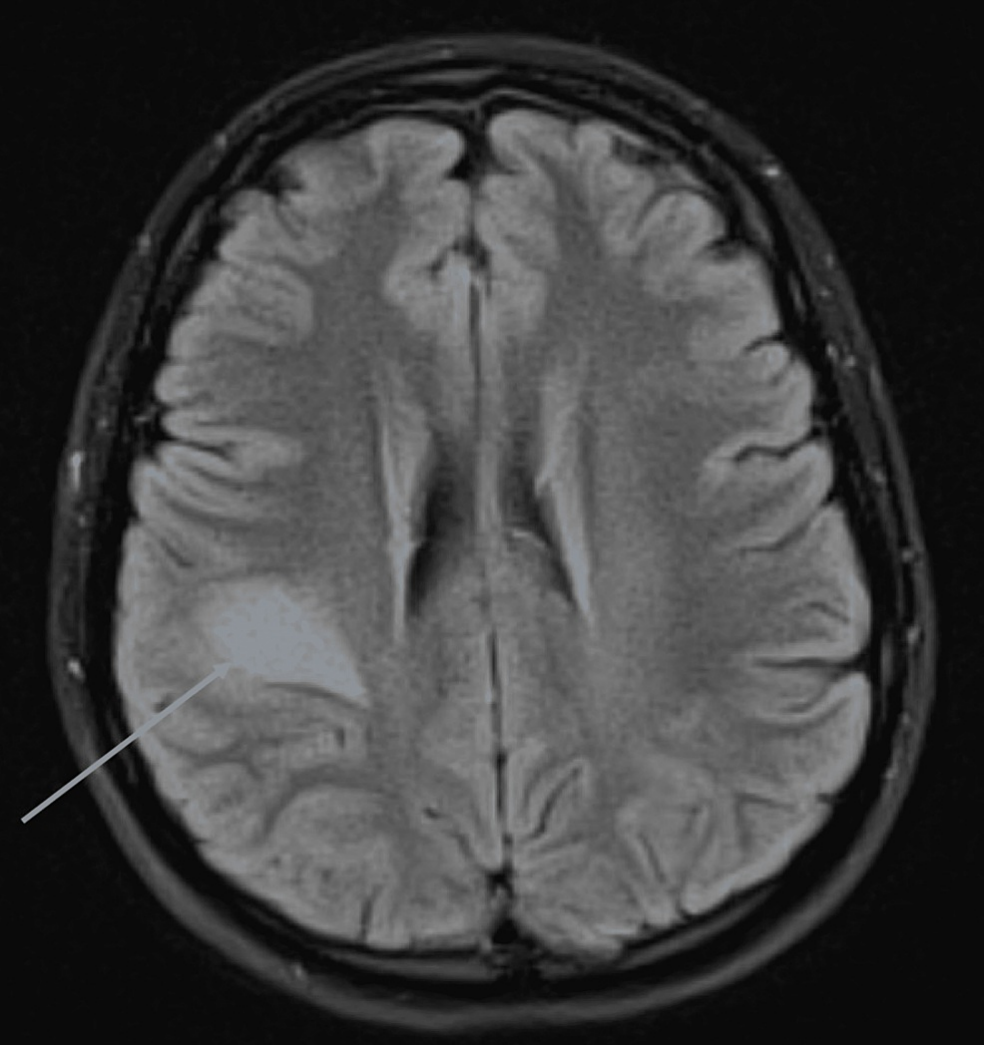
Brain MRI with contrast demonstrating a subacute ride-sided frontal parietal infarction (arrow), exhibiting diffusion restriction, FLAIR, DWI hyperintensity FLAIR, fluid-attenuated inversion recovery; DWI, diffusion-weighted imaging
